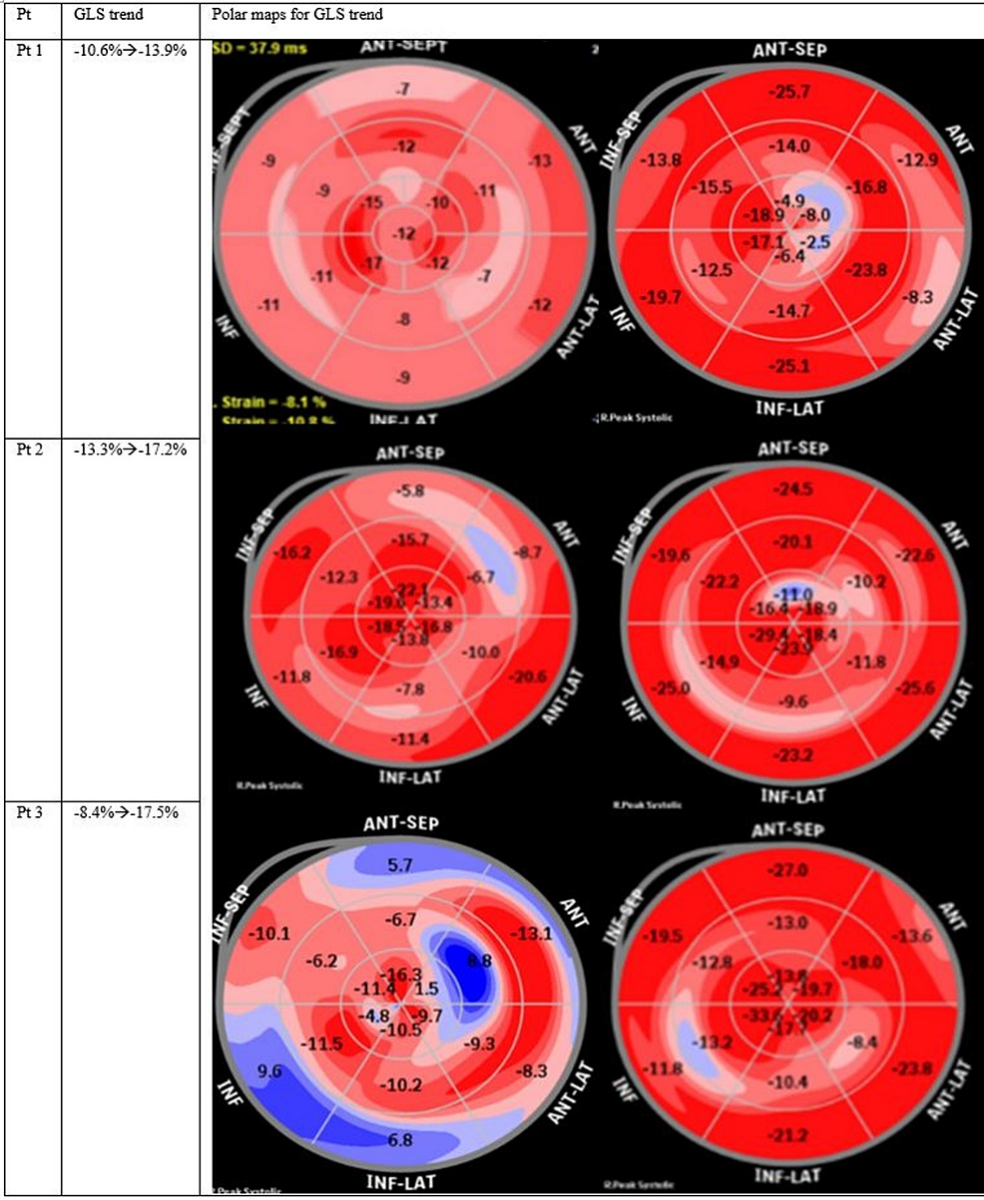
Trend of Global Longitudinal Strain derived by speckle tracking echocardiography. Pt: Patient; GLS: Global Longitudinal Strain
